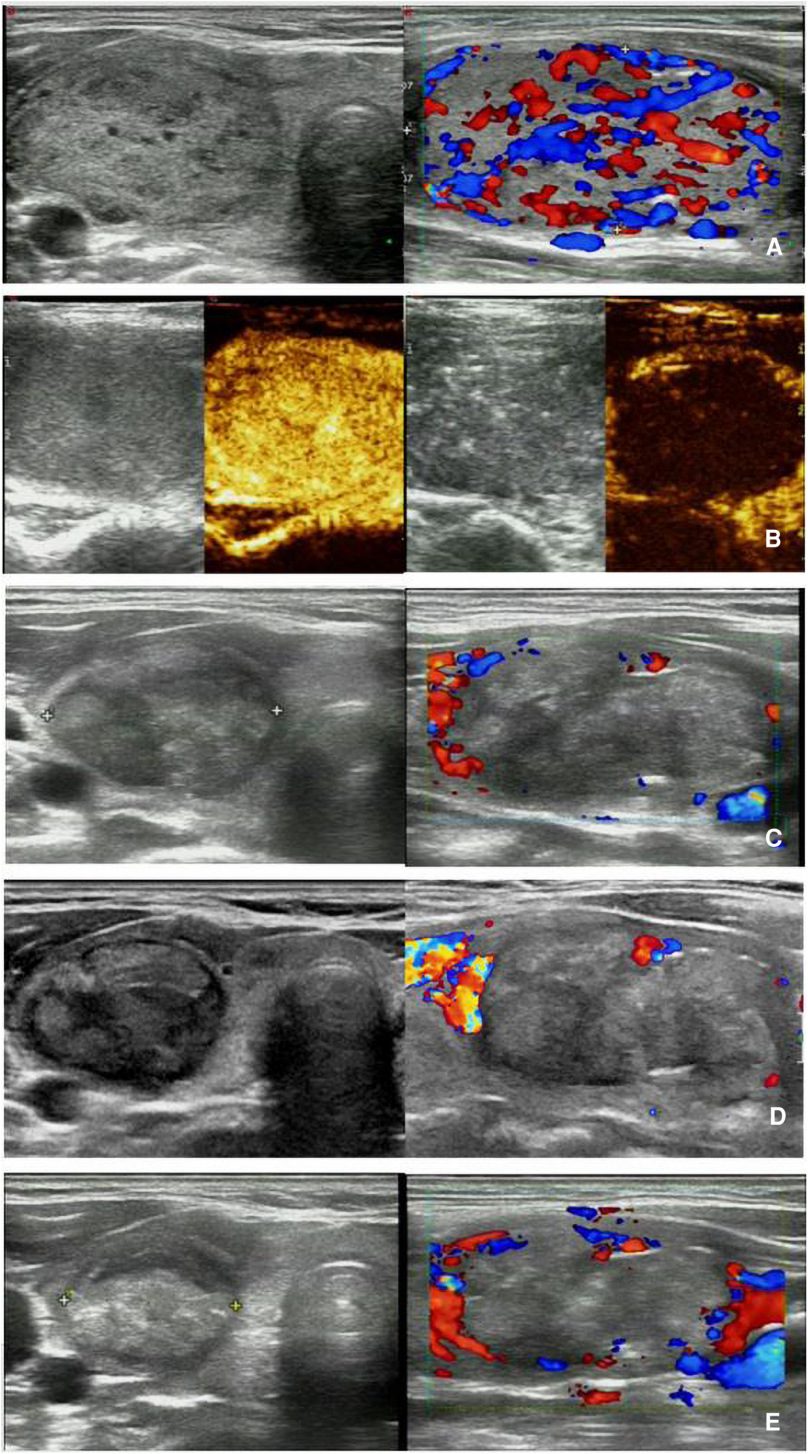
MWA-treated BTN with HT in a 37-year-old woman. (A) Pre-MWA transverse grayscale ultrasound (left) and longitudinal CDFI (right) demonstrate a nodule with regular shape and clear margin, abundant blood flow, size of 48 × 31 × 33 mm, volume of approximately 17.93 ml, and pathologically confirmed as BTN by needle biopsy; (B) Pre-MWA CEUS (left) shows the nodule with uniform hyperenhancement, and post-MWA CEUS (right) shows the nodule without enhancement; C-E. Post-MWA at 1 month (C), 3 months (D), and 6 (E) months after MWA; transverse grayscale ultrasound (left) and longitudinal CDFI (right) shows that the nodules gradually decreased size, with sizes of 40 × 26 × 20 mm, 34 × 22 × 18 mm, and 32 × 18 × 16 mm, respectively, volumes of approximately 10.90 ml, 7.06 ml, and 4.83 ml, respectively, and VRR of 39.21%, 60.62%, and 73.06% respectively. MWA, microwave ablation; BTN, benign thyroid nodule; HT, Hashimoto's thyroiditis; VRR, volume reduction ratio; CDFI, Color Doppler Flow Imaging; CEUS, contrast-enhanced ultrasound.
